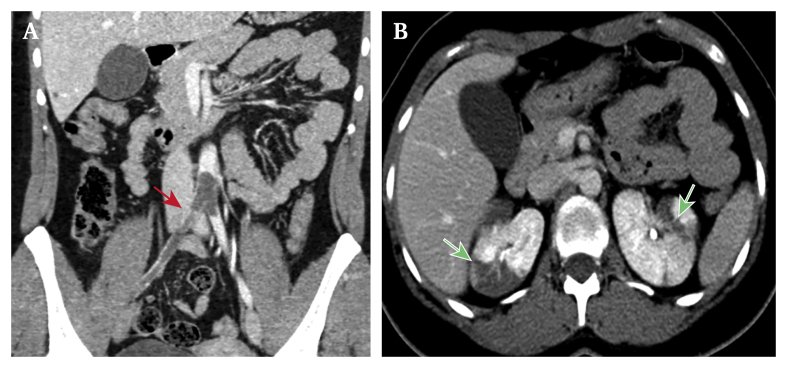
Computed tomography angiography images. (A) Evidence of a saddle embolus of the aortic bifurcation (red arrow) and occlusion of the right iliac axis. (B) Visceral involvement with multiple focal, wedge shaped renal parenchymal defects involving both the cortex and medulla and extending to the capsular surface (green arrows).
